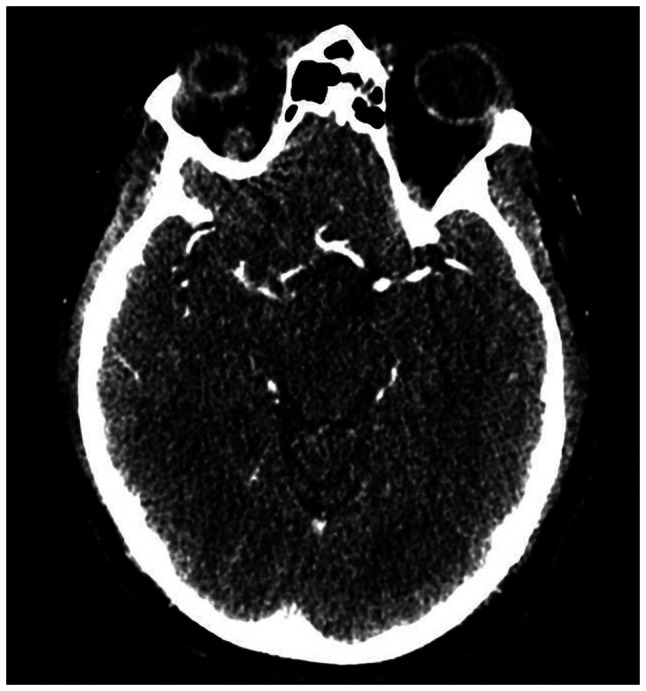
Computed tomography angiography scan.
